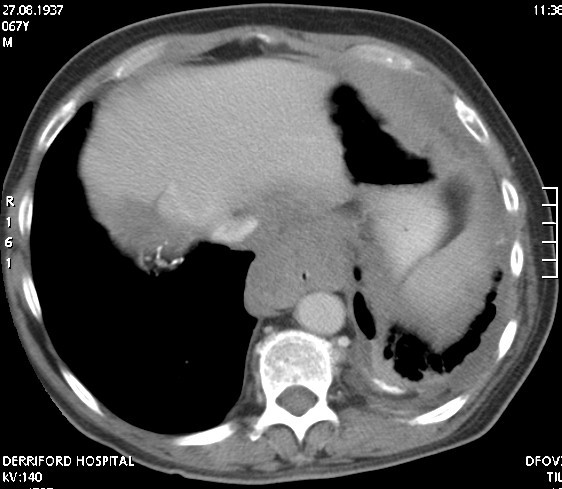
CT scan showing extensive mesothelioma of the chest wall and esophagus.
